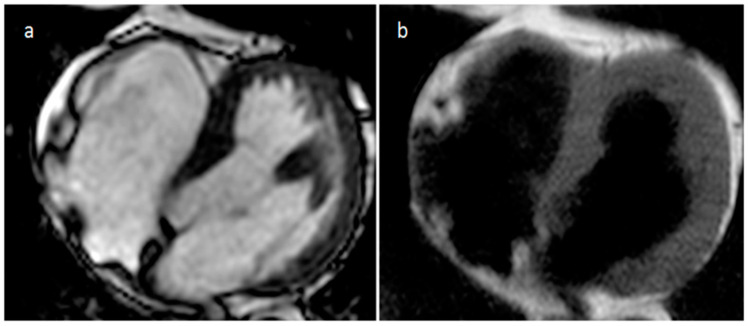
Axial cine MR sequences (a) and T1-weighted images (b) in a patient with prominent crista terminalis.
